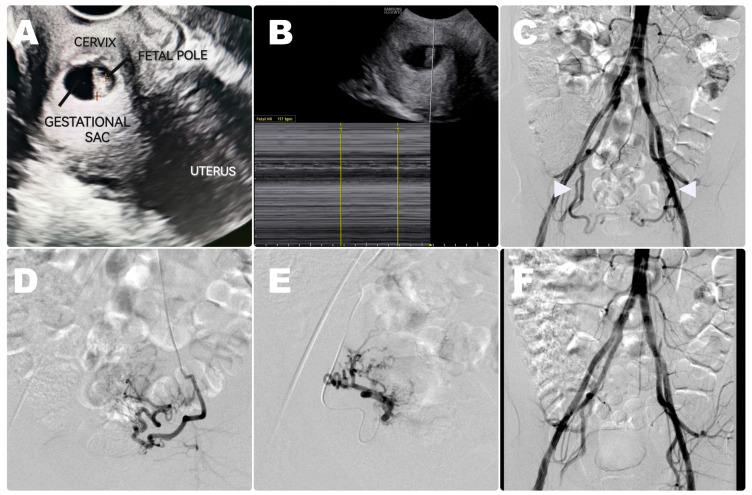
Case of a 33-year-old patient presenting with vaginal bleeding. Transvaginal ultrasound examination suggested a diagnosis of cervical pregnancy (A). The presence of a fetal heart rate was confirmed on ultrasound examination (B). The patient qualified for endovascular treatment. The initial aortography showed blood supply originating from uterine arteries (white arrows) with no collateral flow from ovarian arteries (C). Afterward, selective catheterization of uterine arteries was performed, and embolization with methotrexate and gelfoam was carried out (D,E). The final angiography showed no flow to the gestational sac (F).
